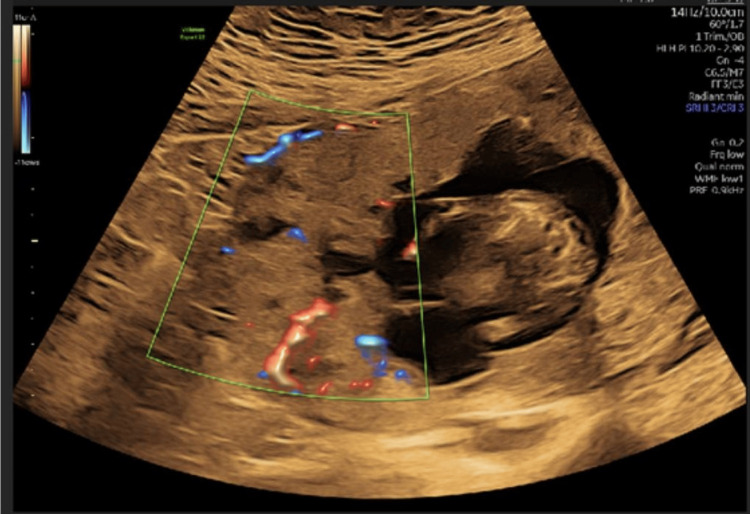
First-trimester ultrasound demonstrates the lack of interface between the placenta and the myometrium, and a serosa composed of abundant placental lakes and increased vascularity.
